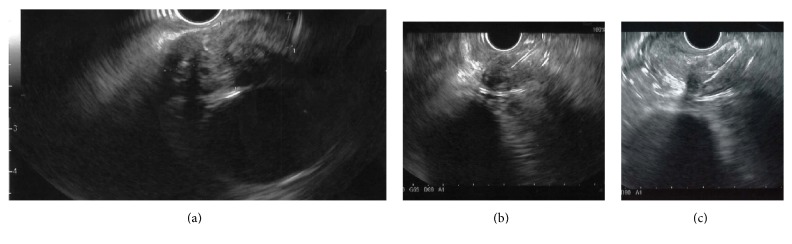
Subcarinal lymphadenopathy on transesophageal EUS (a). EUS-FNA attempt with a 19-gauge needle (b). EUS-FNA attempt with a 22-gauge needle (c).
